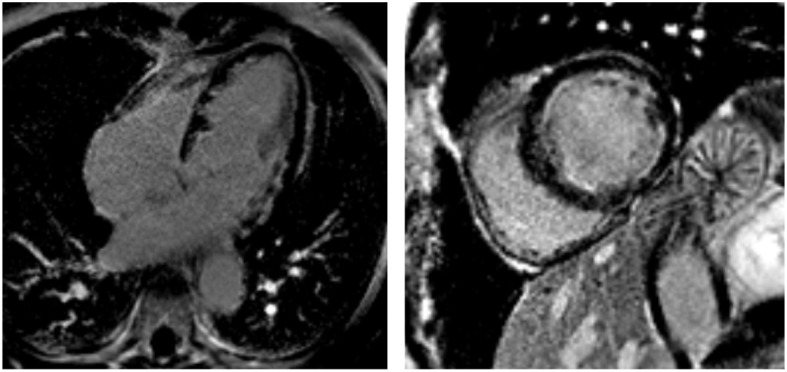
Loeffler cardiomyopathy. LGE sequences performed 5 months after the onset of eosinophilic myocarditis show a circumferential pattern of subendocardial fibrosis (hyperintense areas) in the apical four-chamber view (left) and mid-ventricular short-axis view (right). The subendocardial fibrosis does not follow a coronary distribution and produces a “V-shaped” appearance of the apical ventricles in the apical four-chamber view.
